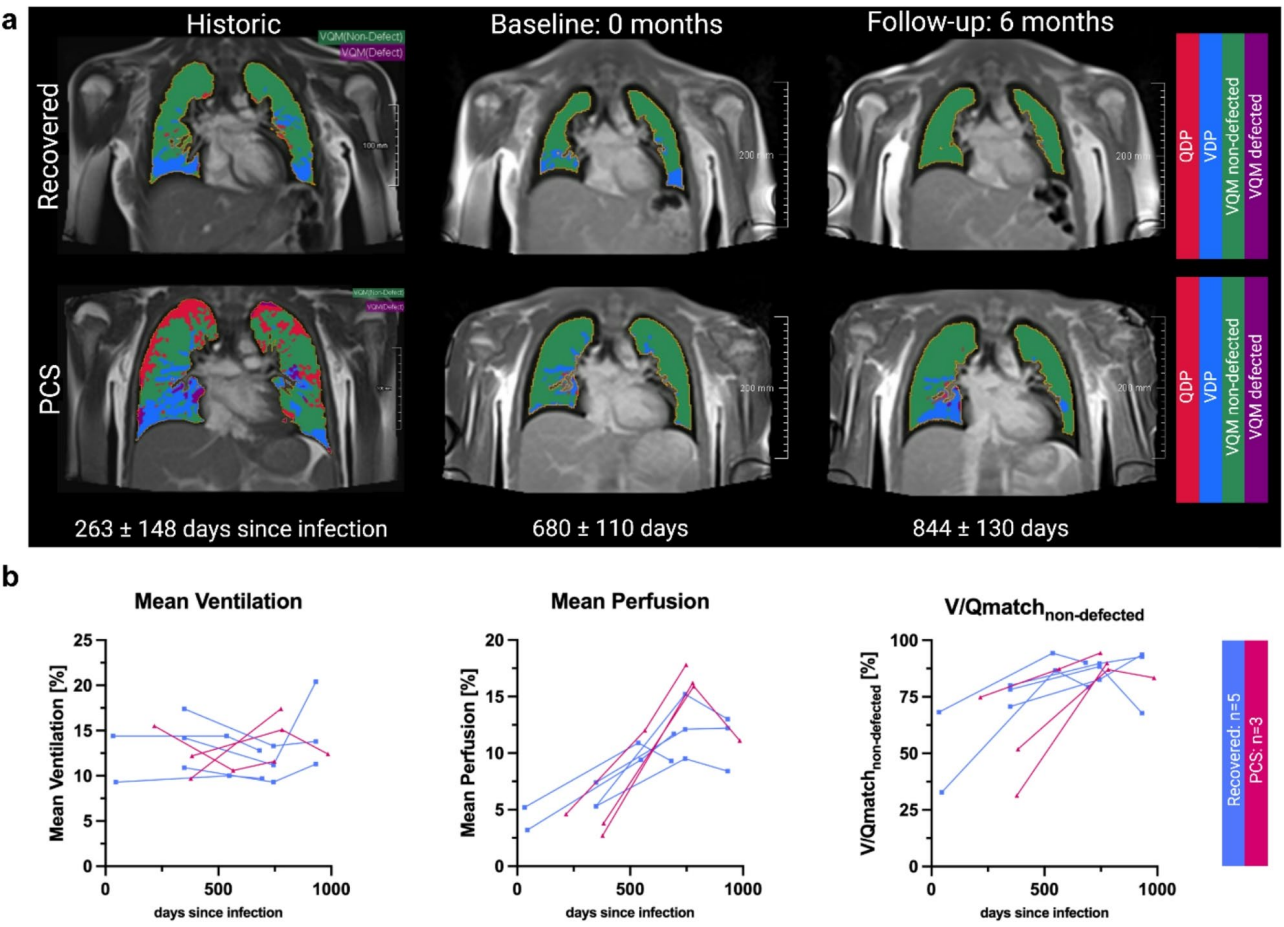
Representative long-term PREFUL MRI assessments. a Representative images of V/Qmatch derived from PREFUL MRI of a recovered and a PCS participant followed over 844 days after initial infection. Historic data is derived from a preliminary study [29]. Provided scales are 100mm for historic and 200mm for baseline and follow-up. b Connected dot plots show trends for ventilation, perfusion and V/Qmatchnon-defected in recovered and PCS cohorts. Rec = Recovered, PCS = Post-COVID-19 syndrome, PREFUL MRI = Phase-resolved functional lung low-field magnetic resonance imaging, QDP = Perfusion defect percentage, VDP = Ventilation defect percentage, V/Qmatch = Ventilation-perfusion match
